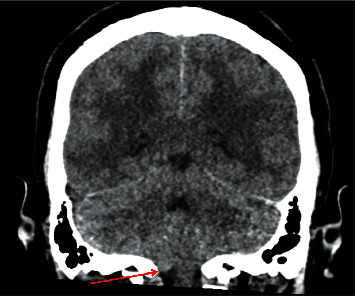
Computed Tomography (CT) showing cerebellar tonsil herniation (red arrow).
